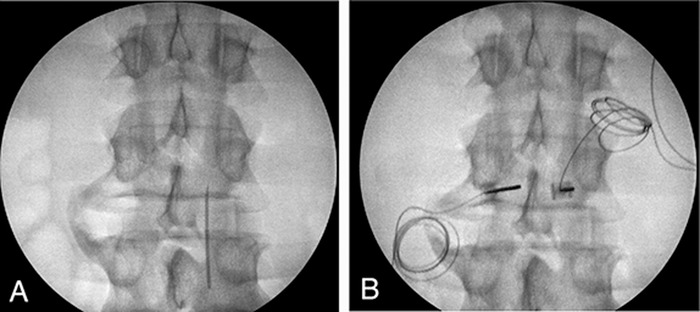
(A) Radiographic image identifying target placement for the introducer with a pointer for the SPRINT peripheral nerve stimulation device at the level of L3, targeting the median nerves for the patient described in case 5. (B) Radiographic image of the introducer placement for the SPRINT peripheral nerve stimulation device at the level of L3 bilaterally, targeting the median nerves for the patient described in case 5.
